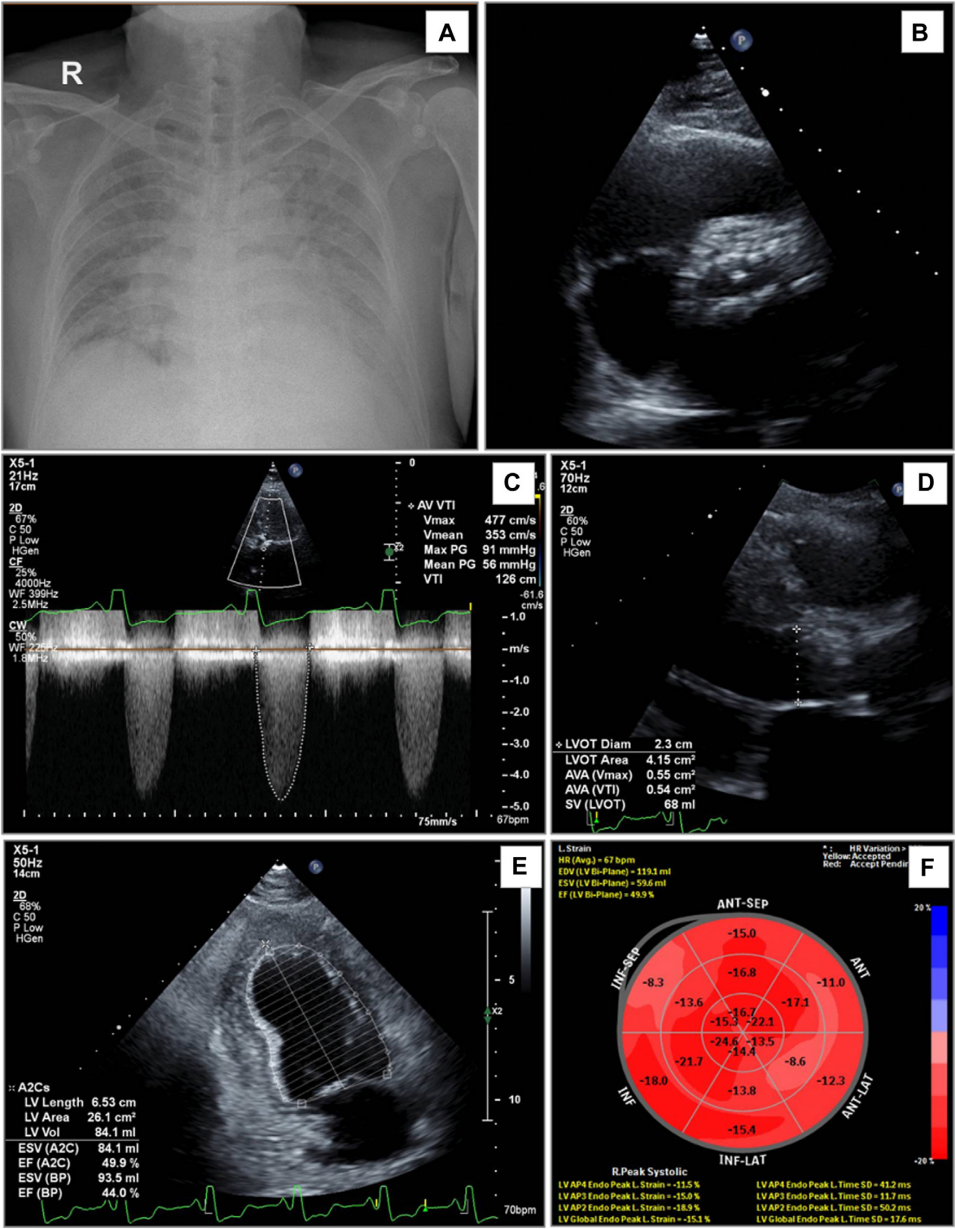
Chest Radiography and Transthoracic Echocardiography (A) Chest radiography demonstrates bilateral pulmonary congestion. (B to F) Transthoracic echocardiography reveals a severely calcified trileaflet aortic valve with significant stenosis (maximum velocity [Vmax], 4.77 m/s; aortic valve area [AVA], 0.54 cm^2^), mildly reduced left ventricular (LV) systolic function (ejection fraction, 44%), and mild left ventricular strain reduction. ANT = anterior; AV = aortic valve; C = compression; CF = color flow; CW = continuous wave; EDV = end-diastolic volume; EF = ejection fraction; ESV = end-systolic volume; H = harmonics; HR = heart rate; INF = inferior; LAT = lateral; LVOT = left ventricular outflow tract; P = persistence; PG = pressure gradient; SEP = septal; SV = stroke volume; Vmean = mean velocity; VTI = velocity time integral; 2D = 2-dimensional.
